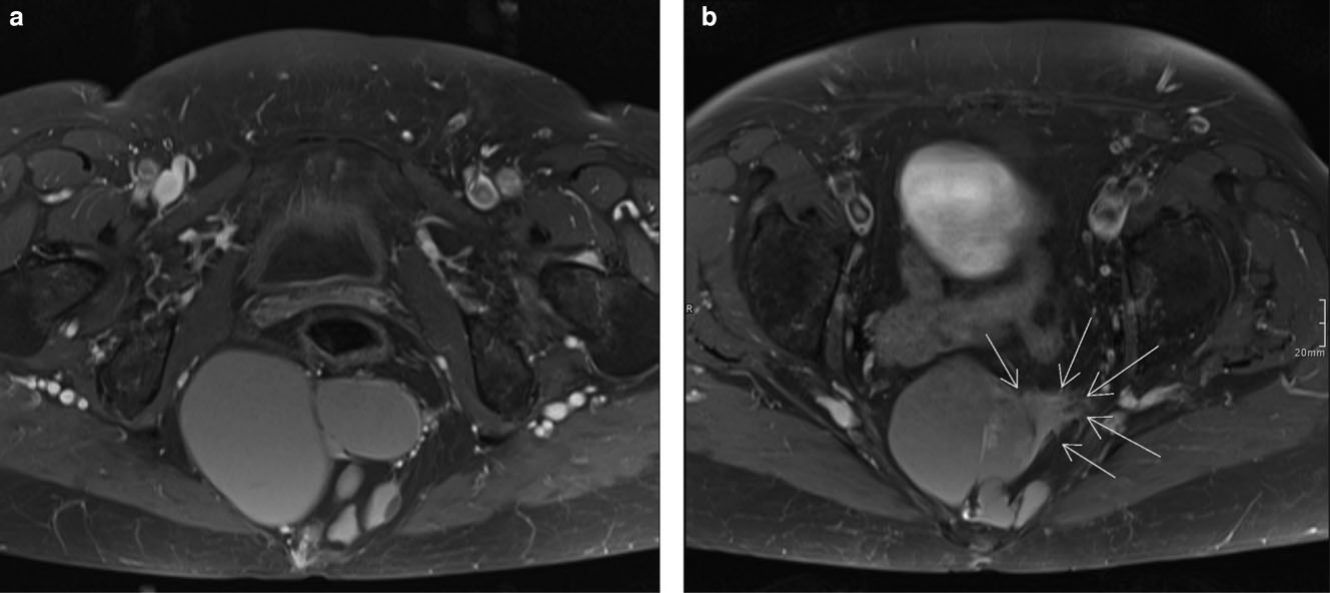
Transformation of a tailgut cyst from a cystic appearance (a) to a mixed heterogenous component marked with arrows (b) on surveillance MRI axial images.
